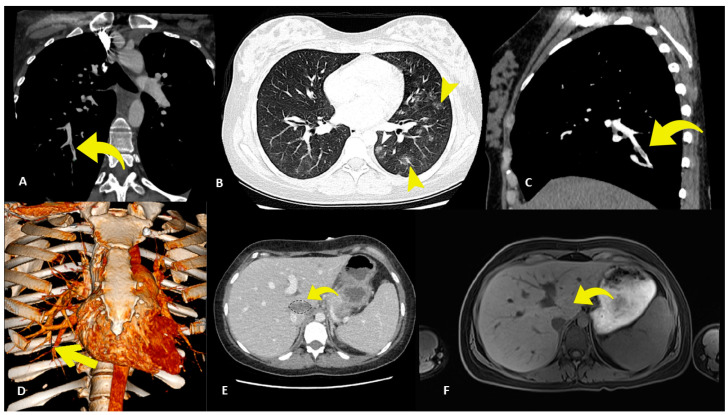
(A) Angiography CT scan showing the presence of some filling defects (yellow curved arrow) in a segmental branch of the right lower lobe of the lung. (B) Lung CT scan with axial scanning showing the presence of multiple ground-glass opacities in the lower lobes (small yellow arrowhead). (C) Multiplanar reconstruction of angiography CT scan highlighting some filling defects (yellow curved arrow) in a segmental branch of the right lower lobe of the lung. (D) Volume-rendering reconstruction highlighting the location of a filling defect in a branch of the right lower pulmonary artery (yellow straight arrow). (E) Contrast-enhanced CT image revealing a hypodense formation anterior to the inferior vena cava. (F) MRI image demonstrating disappearance and healing of the hepatic lesion.
